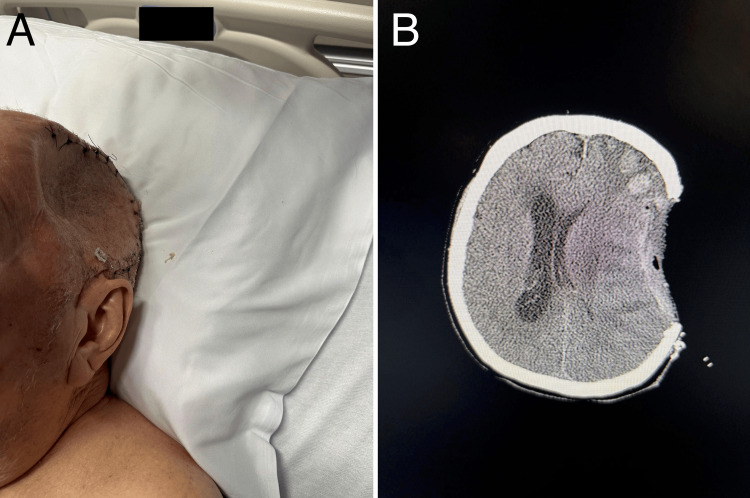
Acute Sinking Skin Flap Syndrome Patient exhibits cranial depression consistent with Sinking Skin Flap Syndrome (SSFS) at the craniectomy site (A). Non-contrast CT imaging depicts a 9mm left-to-right midline shift (B).
